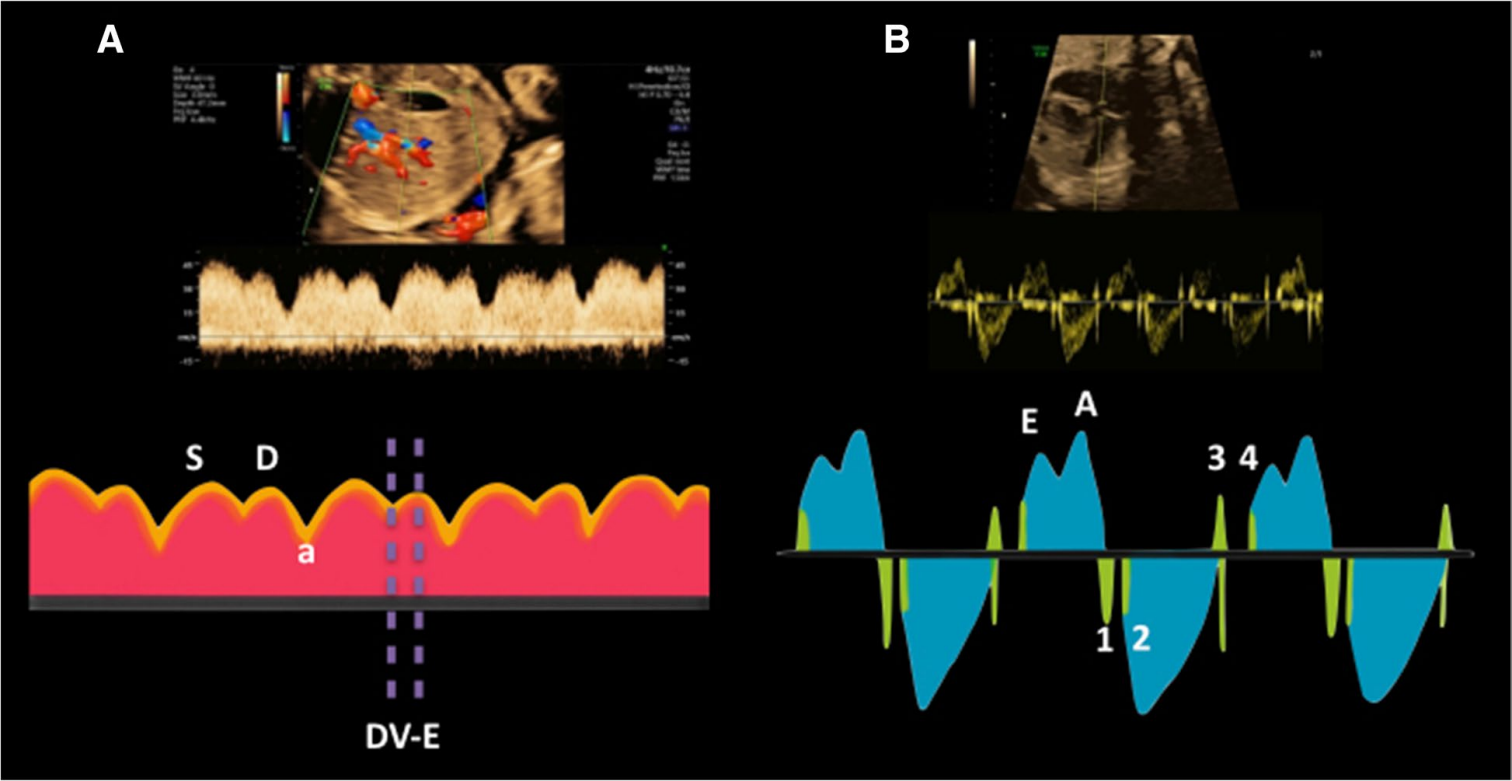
A Early diastolic filling time measured in ductus venosus Doppler waveforms (DV-E) (S = systolic wave, D = diastolic wave, and a = atrial wave); B Left myocardial performance index (LV-MPI) Doppler waveforms, 1 = mitral valve closure click, 2 = aortic valve opening click, 3 = aortic valve closure click, and 4 = mitral valve opening click (E = peak velocity in early diastole, A = peak velocity in late diastole by atrial contraction)
